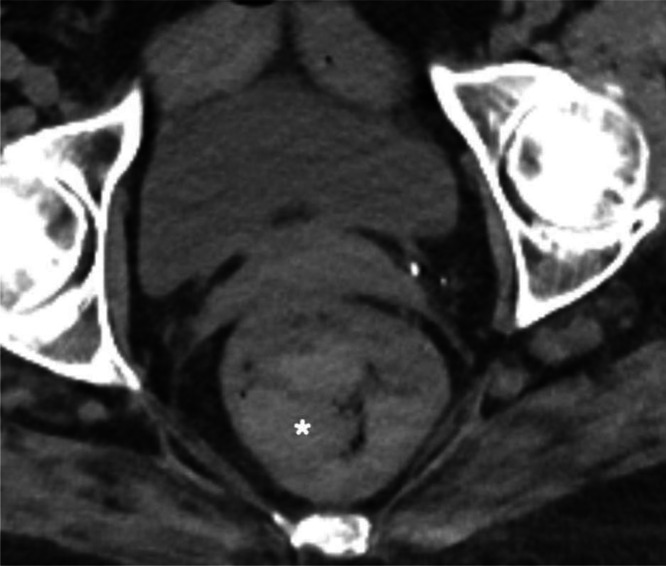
Contrast-enhanced CT, axial pelvis, showing blood clots (asterisk) within the rectal lumen, compatible with active lower gastrointestinal bleeding.
